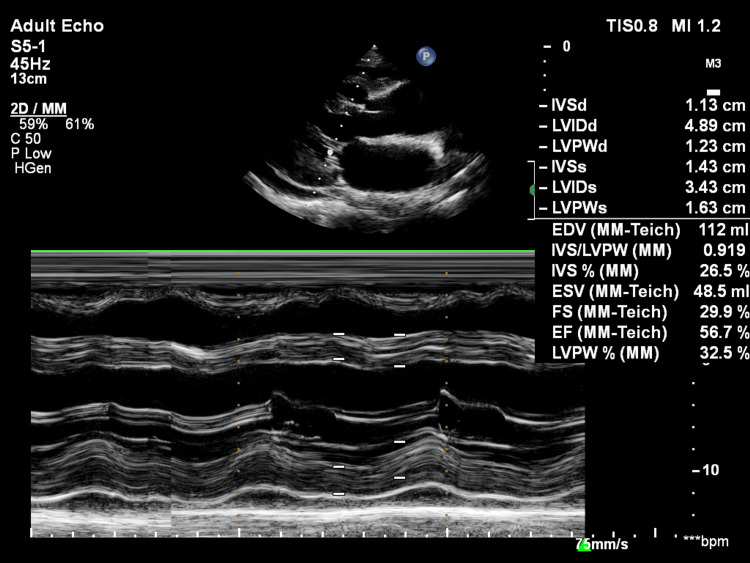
Parasternal long-axis motion mode showing left ventricular ejection fraction estimation. IVSd: interventricular septum diastole; LVIDd: left ventricular internal dimension diastole; LVPWd: left ventricular posterior wall diastole; IVSs: interventricular septum systole; LVIDs: left ventricular internal dimension systole; LVPWs: left ventricular posterior wall systole; FS: fractional shortening; EF: ejection fraction
